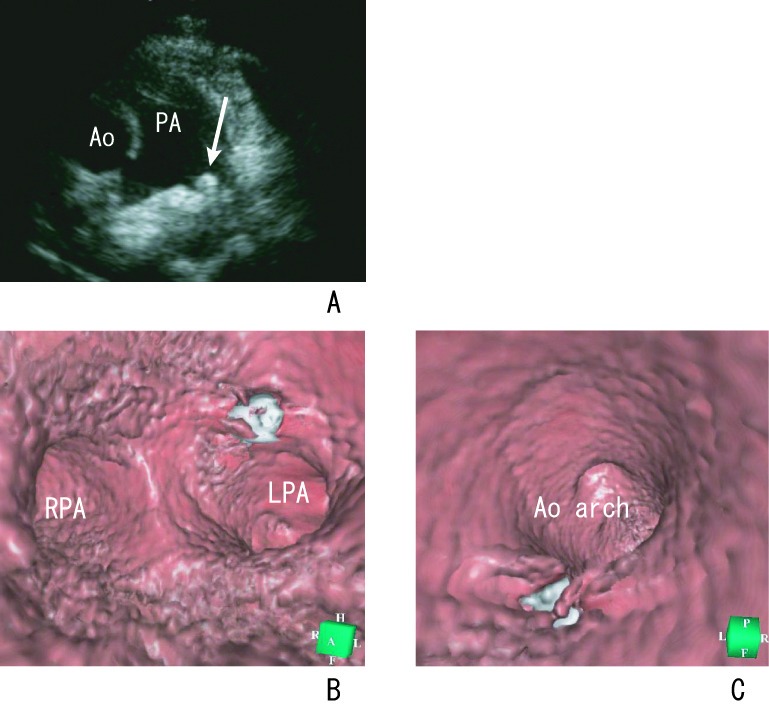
A 6-year-old boy with PDA who underwent transcatheter coil occlusion. (A) Transthoracic echocardiogram shows the coil in the left pulmonary artery. Coil protrusion (arrow) is suspected. Virtual endoscopy shows the location and protrusion of the coil from the pulmonary (B) and aortic (C) sides. The left pulmonary artery is not obstructive. Ao, aorta; LPA, left pulmonary artery; RPA, right pulmonary artery. *Reproduced with permission from Catheterization and Cardiovascular Interventions 2007; 70: 434-439, Wiley-Liss, Inc.*
